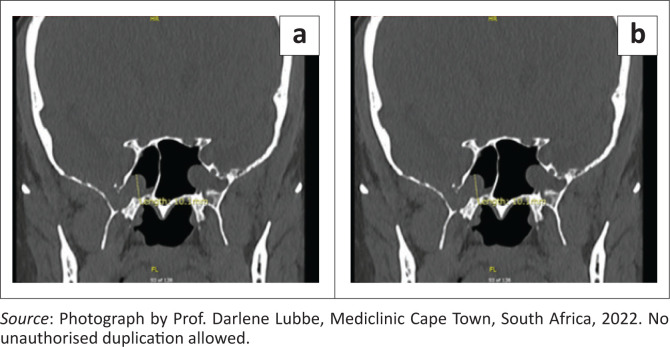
Coronal view of non-contrasted computed tomography scan of paranasal sinus showing bilateral sphenoid sinus encephaloceles.
